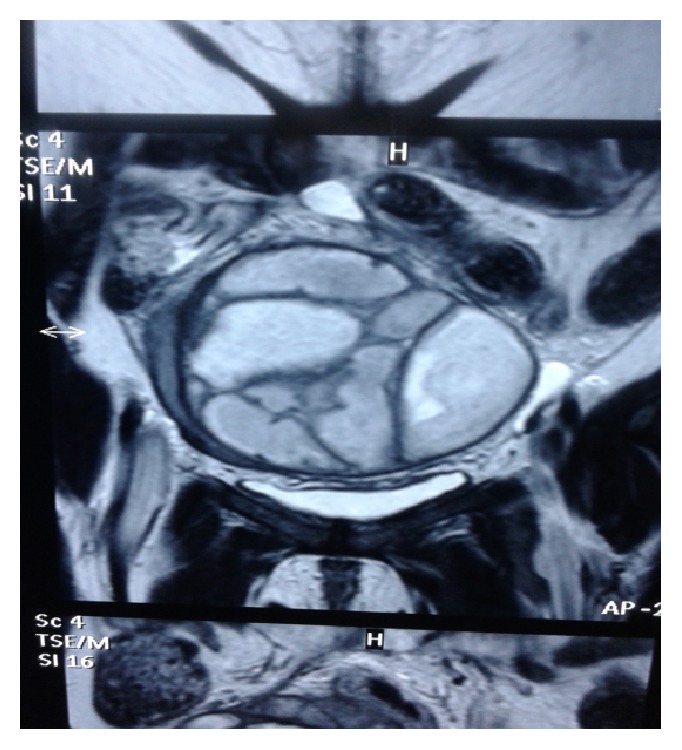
MRI of pelvis showing bilateral tuboovarian masses.
